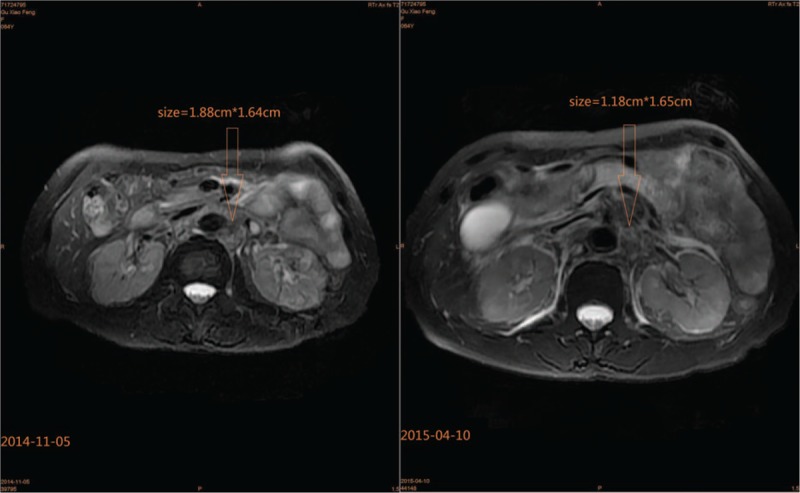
MRI examination of abdomen, showing the mass of retroperitoneal lymph node. Before the targeted therapy of apatinib, the size was 1.88 × 1.64 cm, after 4 months therapy, it reduced to 1.18 × 1.65 cm. MRI = magnetic resonance imaging.
